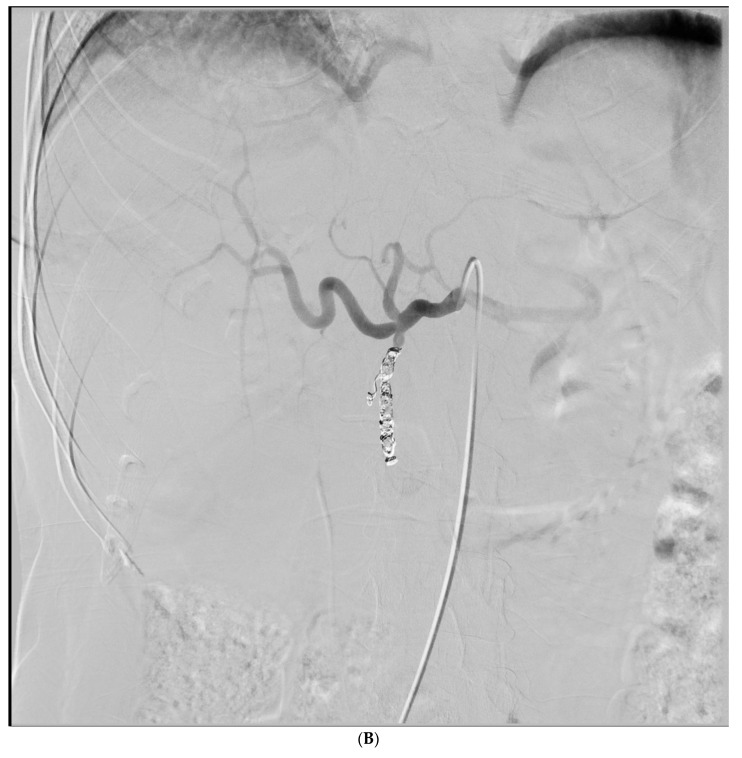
This 48 year old female with metastatic colo-rectal cancer was referred for selective internal radiation therapy, using Yittrium-90. The (A) mapping angiogram showed a trifurcation of the common hepatic artery into the GDA (dashed arrow), left hepatic (curved arrow), and right hepatic (straight arrow) arteries. GDA embolization with this anatomic configuration was felt to be necessary in order to reduce the chances of the non-target duodenal embolization. The (B) GDA was successfully cannulated with a microcatheter, and multiple coils were placed successfully, which embolized this vessel. The Yttrium-90 (Y-90) administration was subsequently performed without clinical or radiographic evidence of non-target embolization to the duodenum.
